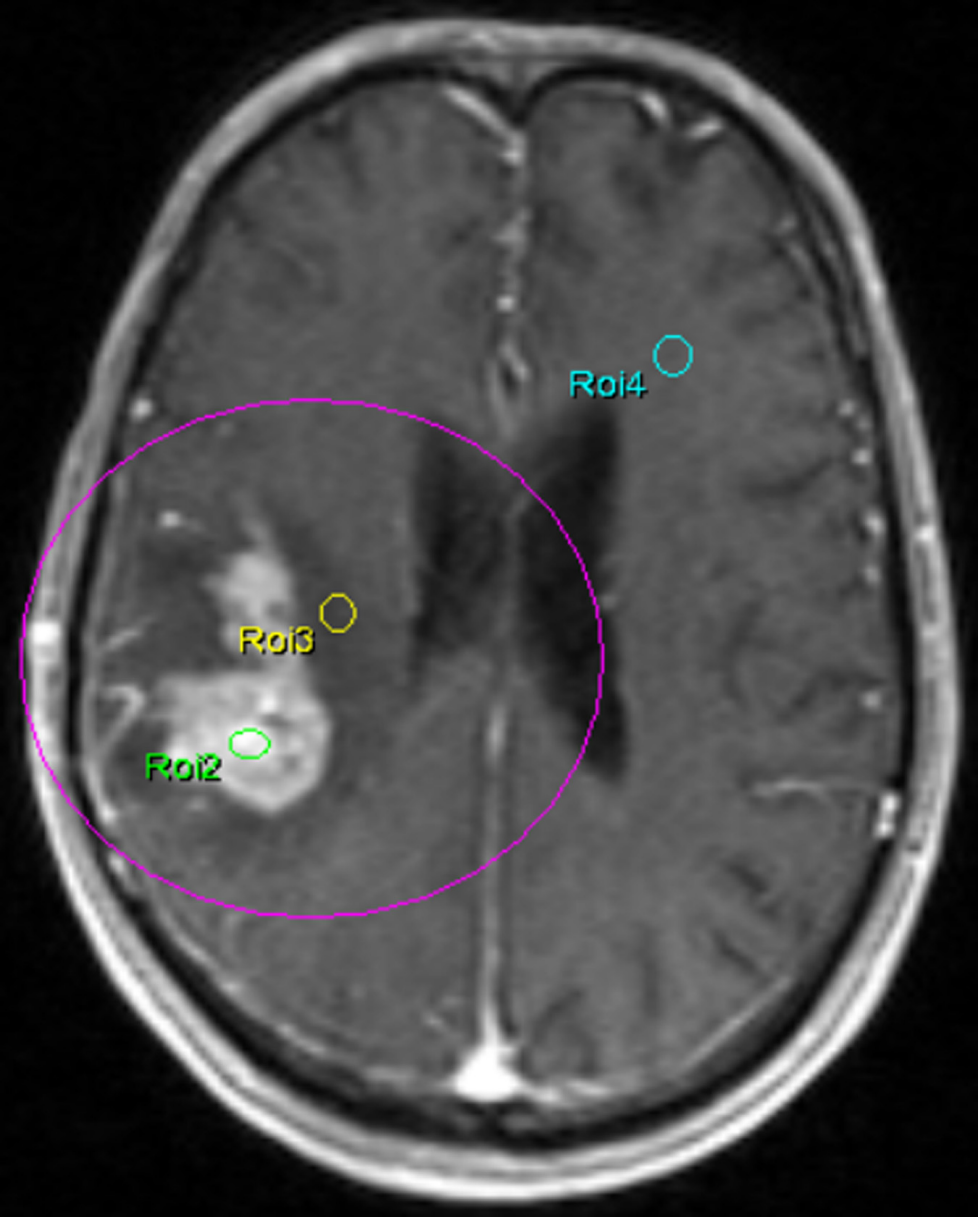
An example of ROIs placing. A 67 years old man with a suspicious high grade glioma in right temporal lobe on T1-weighted contrast-enhanced MRI. ROI 2 and ROI 3 were placed in the tumor parenchyma and peritumoral area, respectively; ROI 4 was put in the contralateral normal hemisphere.
